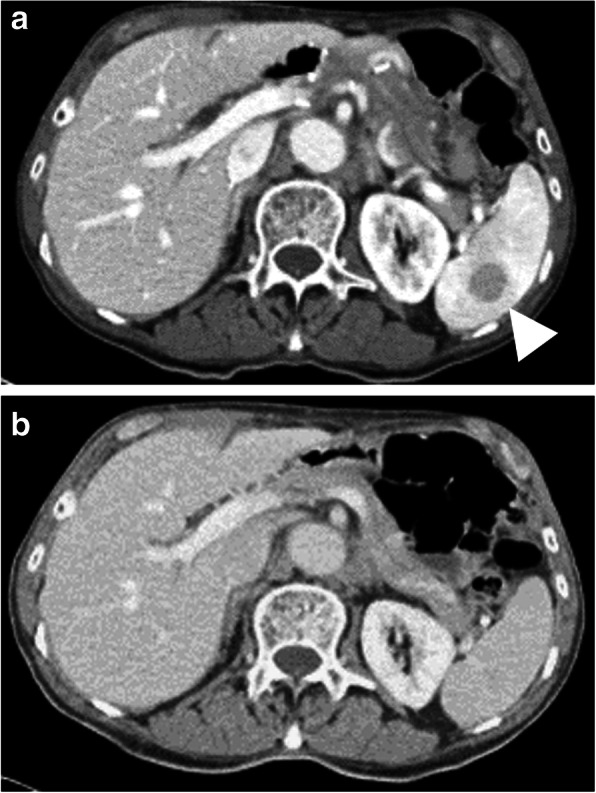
a The CT scan 6 months after gastrectomy shows the appearance of a low-density lesion that is 17 mm in diameter at the spleen. b Preoperative CT scan shows no lesion at the spleen recognized
